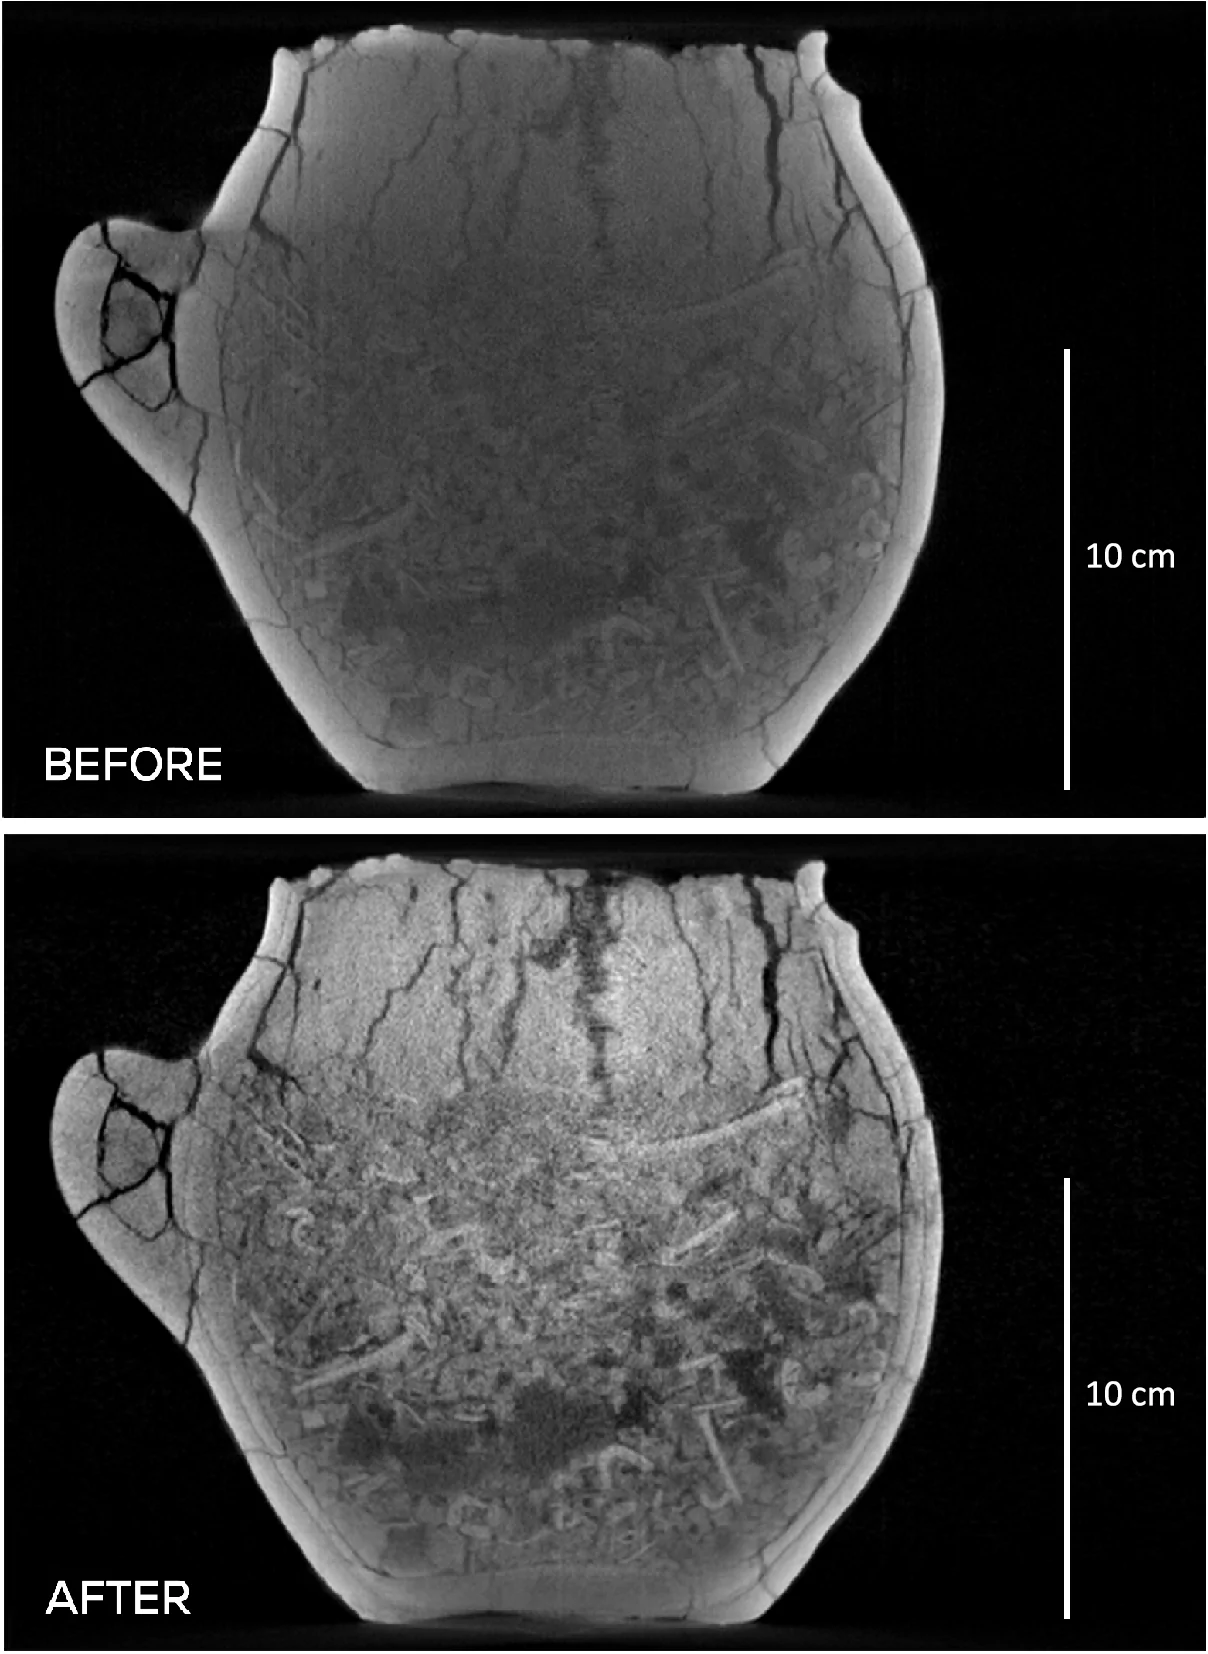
CT images of burial 32 before and after the Beam Hardening correction.
